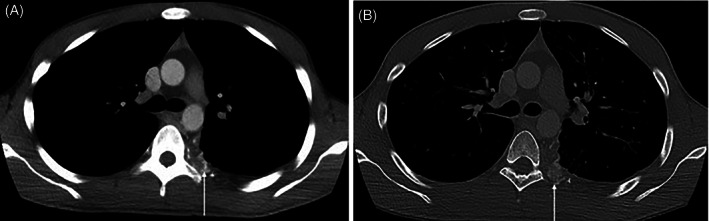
Two‐month follow‐up axial contrast enhanced chest CT mediastinal window image (A), and bone window (B) demonstrates amorphous calcification (white arrow) at postoperative site in left T7 level
